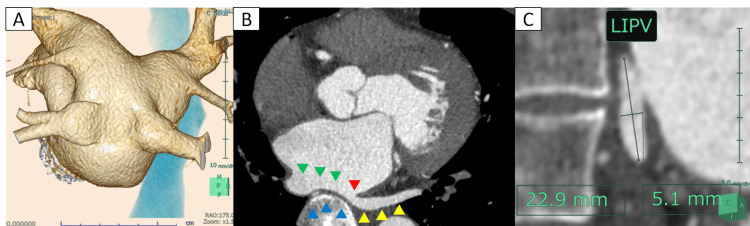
Contrast cardiac CT image. (A) Contrast cardiac CT image of the PA view showing the left atrium and PVs. (B) Contrast cardiac CT image of the axial slice. The blue arrowheads indicate the rim of the spine; the green arrowheads show the common trunk of both inferior PVs; the yellow arrowheads show the left inferior PV; and the red arrowhead shows the narrow ostium of the left inferior PV. The left inferior PV and the common trunk are compressed by the spine. (C) Contrast cardiac CT image of the axial slice to the left inferior PV ostium. The long and short diameters of the left inferior PV ostium measured 22.9 and 5.1 mm, respectively. CT = computed tomography; PA = posterior-anterior; PV = pulmonary vein; LIPV = left inferior pulmonary vein
